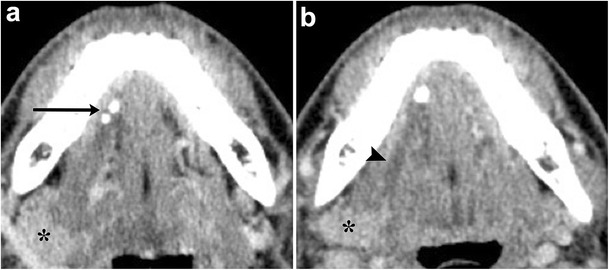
Sialolithiasis with resultant sialadenitis. Axial contrast-enhanced CT images at the level of the submandibular gland demonstrate two well-circumscribed calcifications in the distal right submandibular duct (a, *arrow*). The right submandibular duct is dilated proximal to the stones (b, *arrowhead*). There is enlargement and enhancement of the right submandibular gland (*) compared to the normal left submandibular gland
